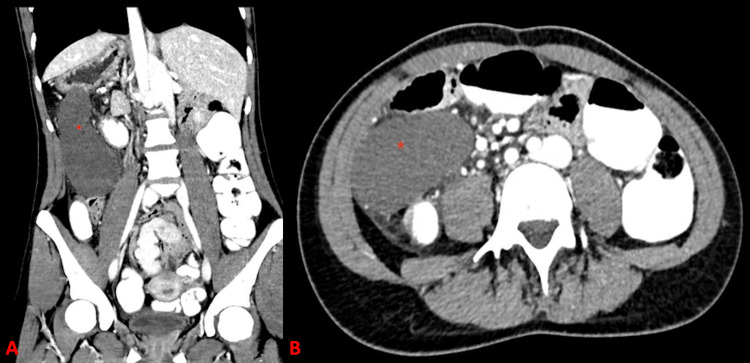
Abdominal CT at the time of admission shows the main spleen with lack of contrast suspected of ischemia (red star) in the coronal (A) and axial (B) views.
